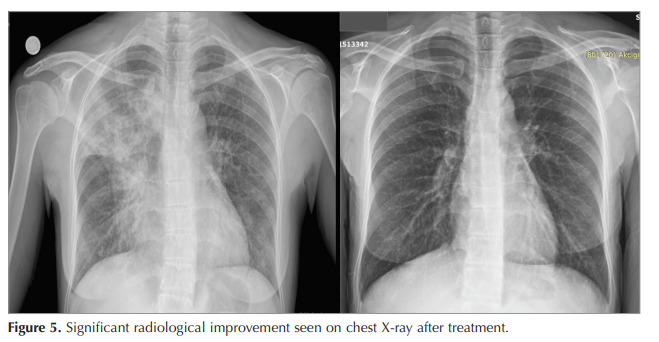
Significant radiological improvement seen on chest X-ray after treatment.
